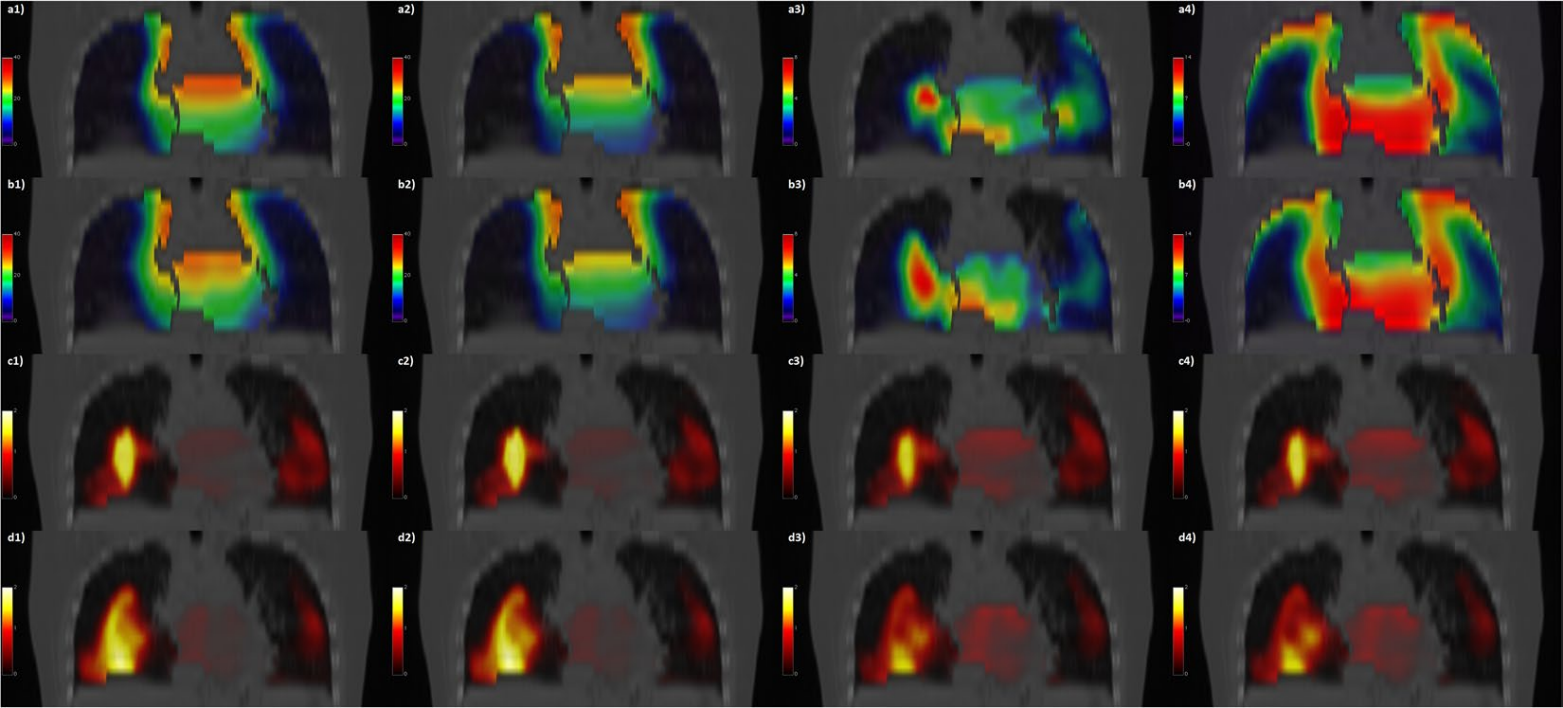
Coronal views of the thorax CT fused with dose-related maps (rows *a* and *b*) and with significance p-maps (rows *c* and *d*). Columns in rows *a* (B-spline) and *b* (Demons) show: 1) mean dose maps (Gy) for patients with RIM; 2) mean dose maps (Gy) for patients without RIM; 3) dose difference between 1) and 2); 4) dose standard deviation map within the cohort. Please note that color maps in columns 3) and 4) have different scales of 1) and 2). Columns in rows *c* (B-spline) and *d* (Demons) show the maps of -Log(*p*) as derived from tests for: 1) GLM on dose; 2) GLM on BED; 3) GLM on dose and age; 4) GLM on BED and age.
